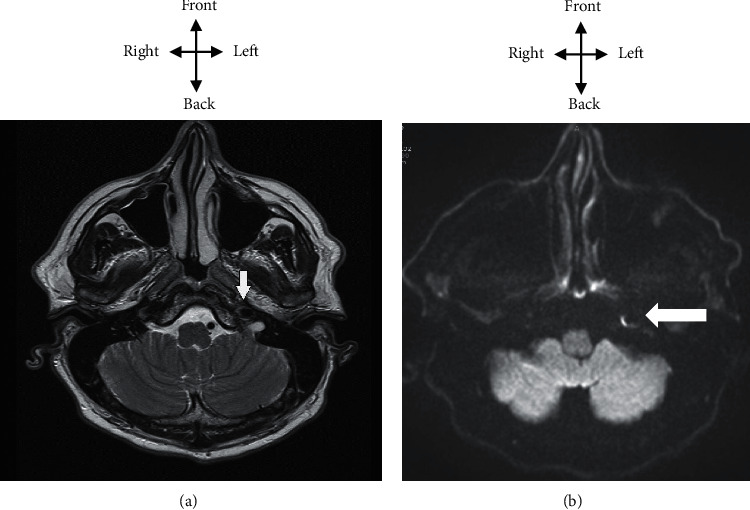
MRI of the neck vessels, T2 TSE axial (a) and diffusion axial (b), aneurysm ectasia of the left internal carotid with parietal irregularity.
